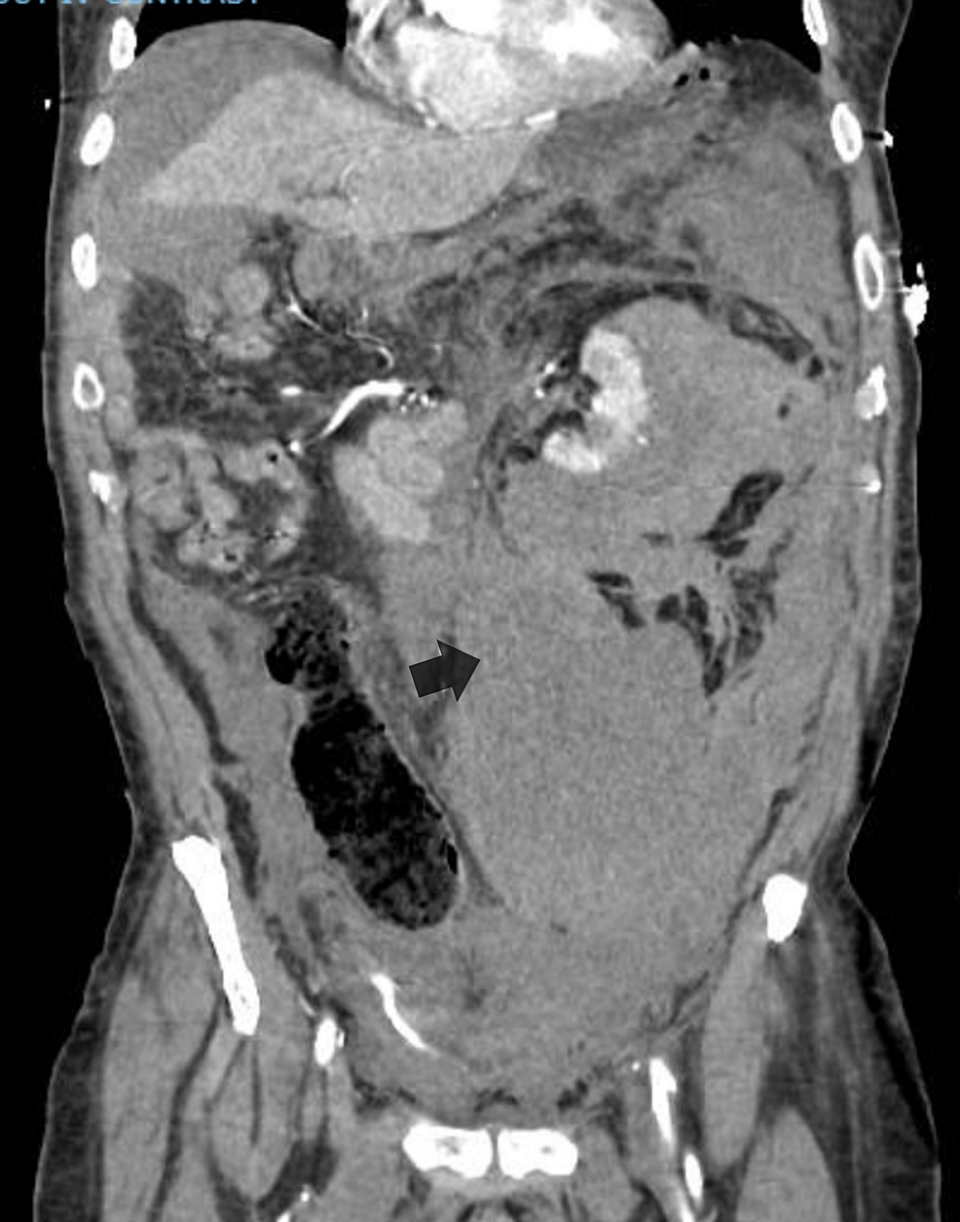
CT scan of the abdomen and pelvis showing massive intra-abdominal bleeding related to renal cell carcinoma (the arrow is pointing toward the intra-abdominal bleeding)
